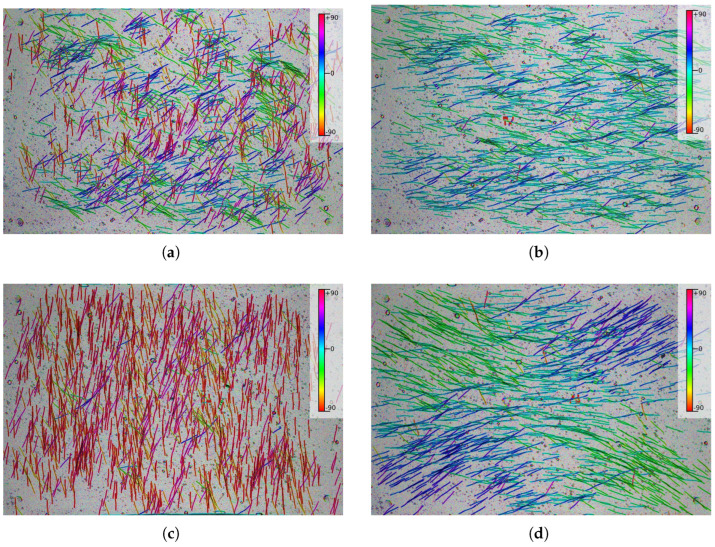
Image analysis of fibers oriented in the ultrasound gel experiments (a) before orientation (random) (b) horizontal (c) vertical (d) cross orientations. Colors represent the fiber angle.
